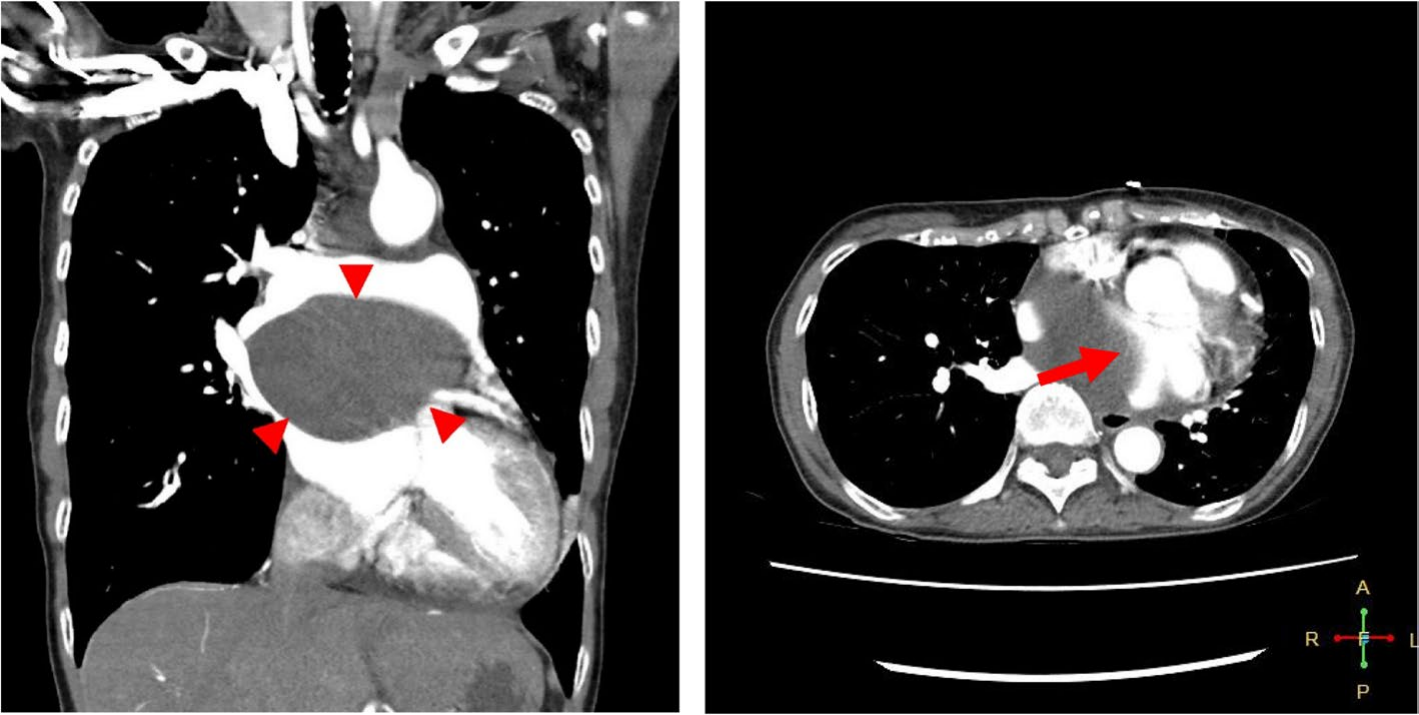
Chest computed tomography images: The cystic lesion (arrowhead) is located between the right main pulmonary artery and the left atrium. The cystic wall strongly adheres to the LA (arrows)
